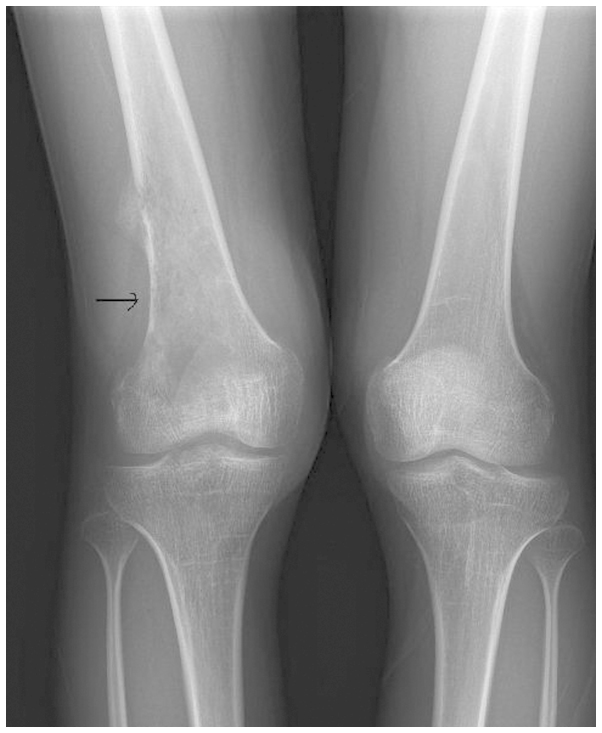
Anteroposterior radiograph of the distal right femur showing a destructive lesion (arrow), with lateral cortical discontinuity and soft-tissue extension.
